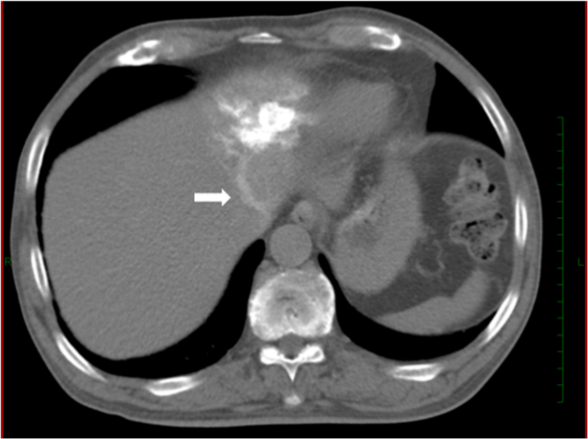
A case of AML with early venous return. CT angiography via the left hepatic artery showed a patchy enhanced tumor in S3 with early venous return to left hepatic vein (arrow).
